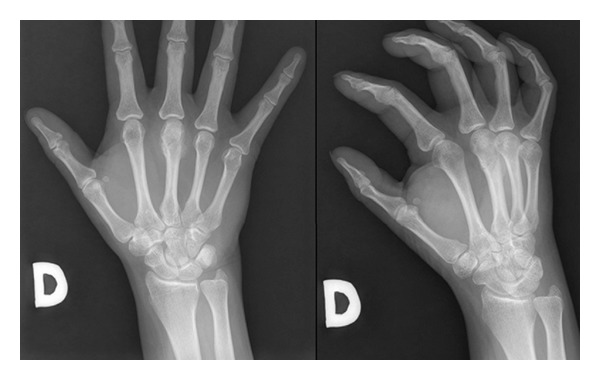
Plain radiograph of the right hand showing a dense mass extending from the dorsal aspect of the third distal metacarpal.
